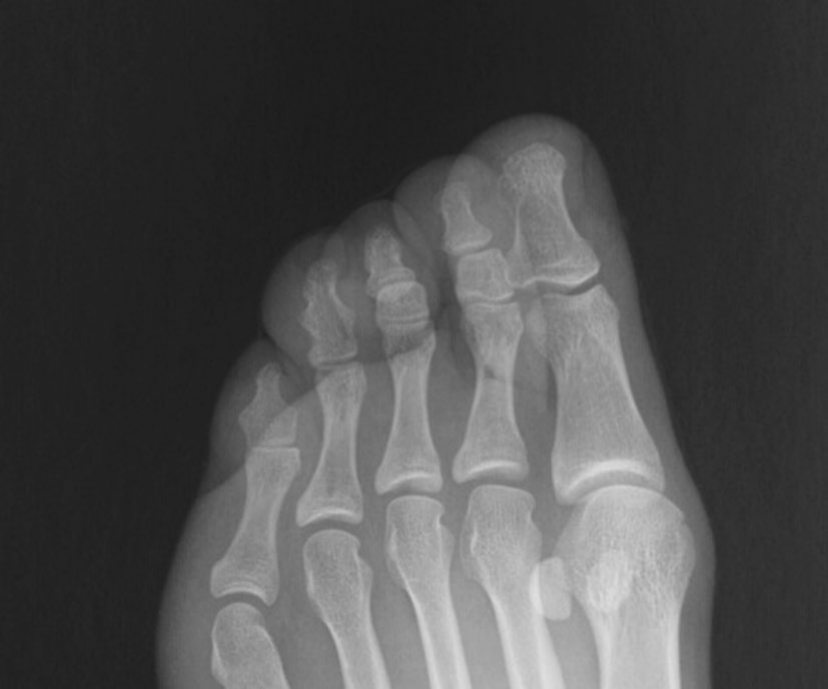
Foot radiograph of an adult shows biphalangeal 4th and 5th toes
